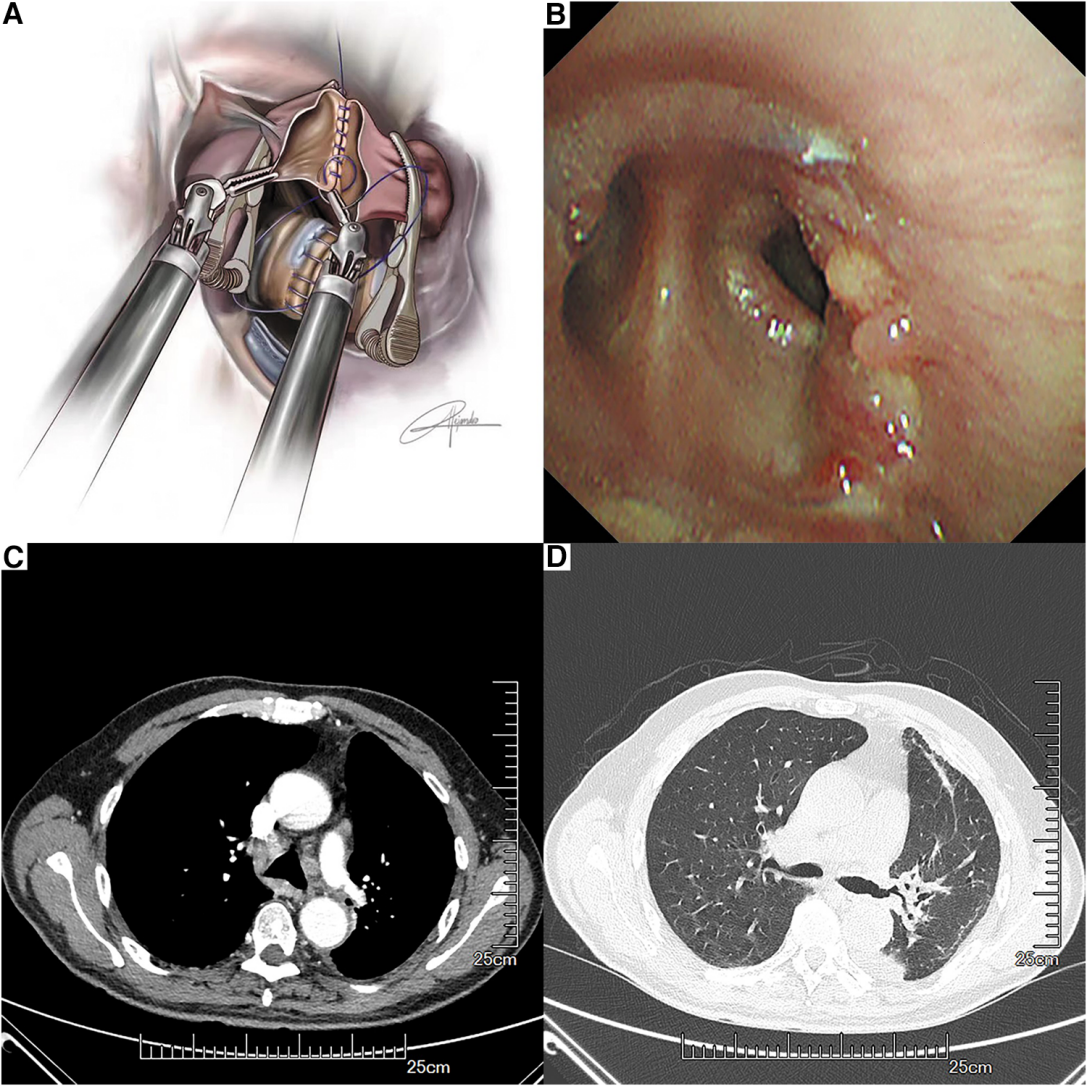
Anastomosis of the left pulmonary artery and the left lower pulmonary artery with a running suture by using bulldog clamps (A). Bronchoscopy findings at the anastomotic site on the 50th postoperative day (B). Recent contrast-enhanced CT (C,D).
